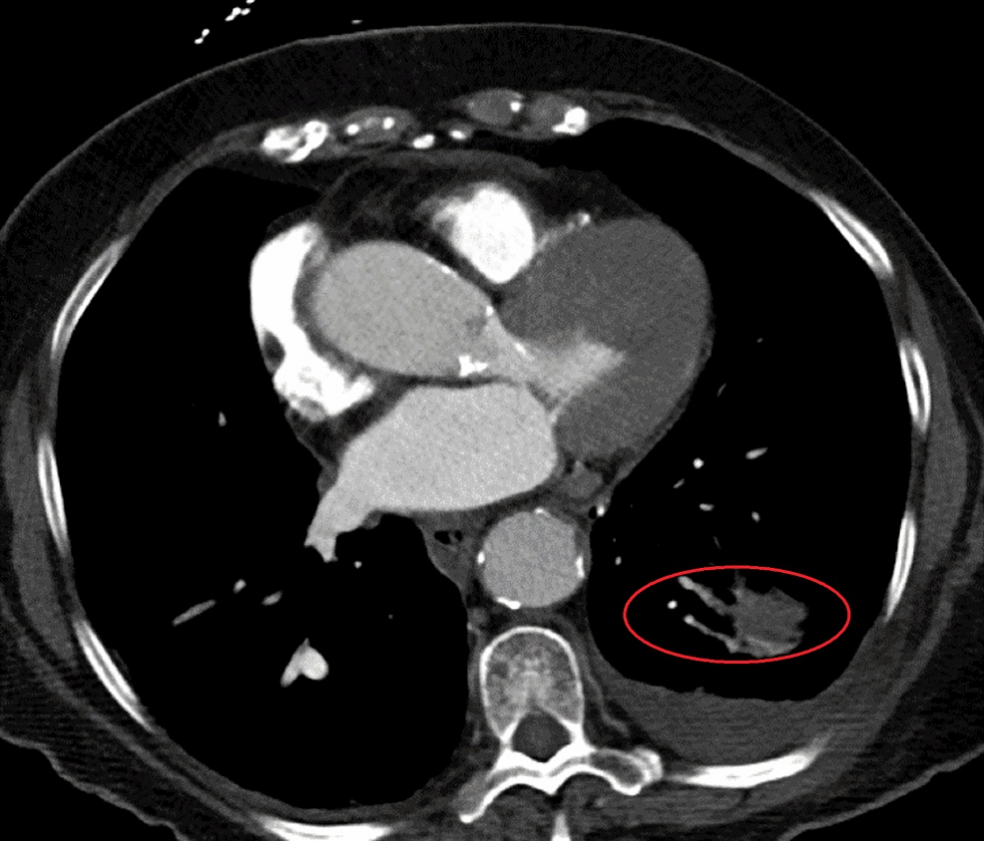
High-resolution coronal CT image demonstrating bronchial thickening and traction bronchiectasis.
